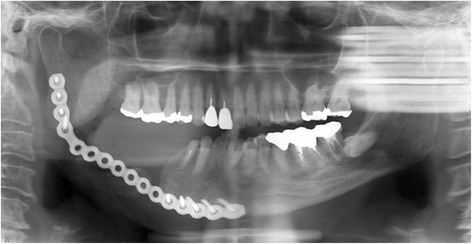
Postoperative panoramic radiograph in case 2. The preoperative relation between the proximal and distal segments and the position of the condylar heads in the temporomandibular fossae were maintained
